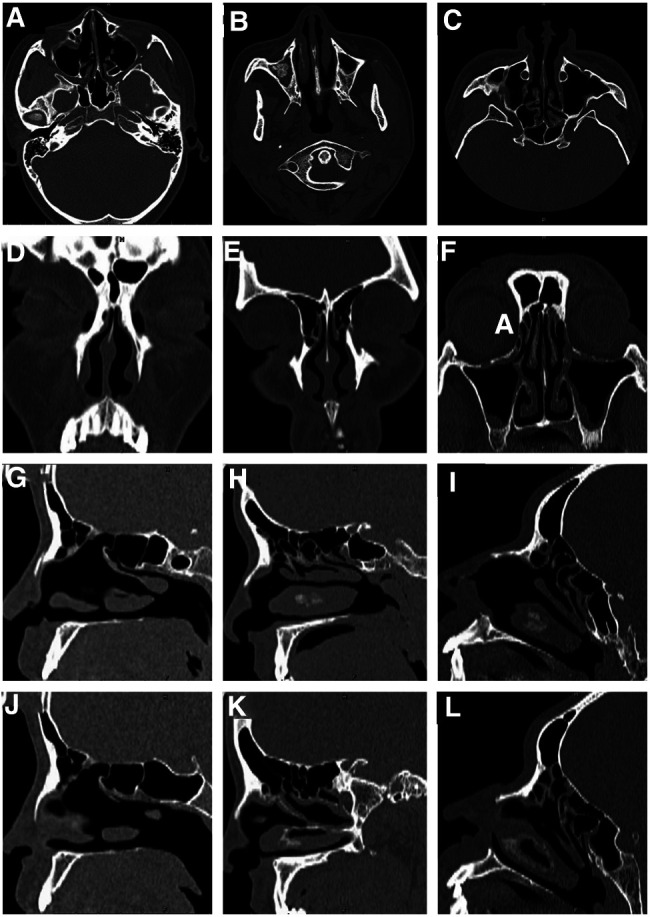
Preoperative thin slice CT examination of paranasal sinus in patients 1, 2 and 3: patients 1 (A, D, G, J), 2 (B, E, H, K), 3 (C, F, I, L), horizontal (A–C), coronal (D–F), and sagittal left (G–I) and right (J–L).
